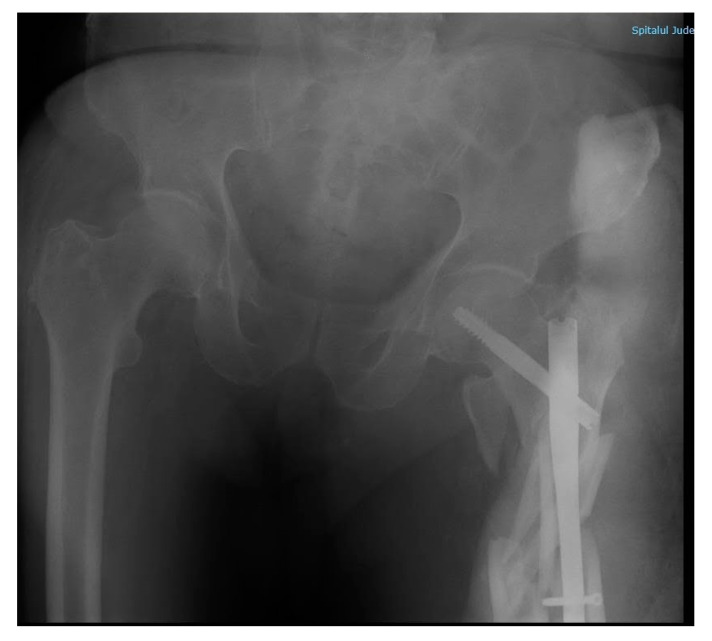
Hip X-ray after 3-m height fall, anteroposterior incidence. Description: male subject. Right hip with minimally narrowed joint space. Left hip in the ⅓ proximal of the femur with osteosynthesis material (femoral rod distally nailed) slightly moved, degraded. Comminuted fracture with bone fragments misaligned in the proximal diaphysis of the left femur, with angulation of fragments. Loss of contour between normally continuous line from medial edge of femoral neck and inferior edge of the superior pubic ramus. Minimally impacted left femoral neck.
